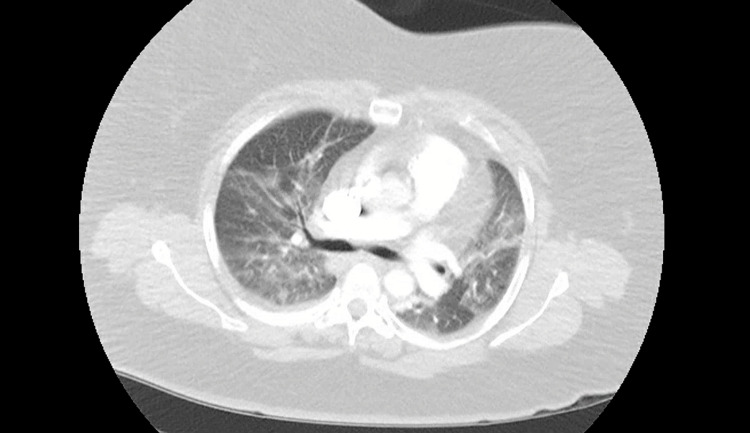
CT Chest 2 Days After Admission
